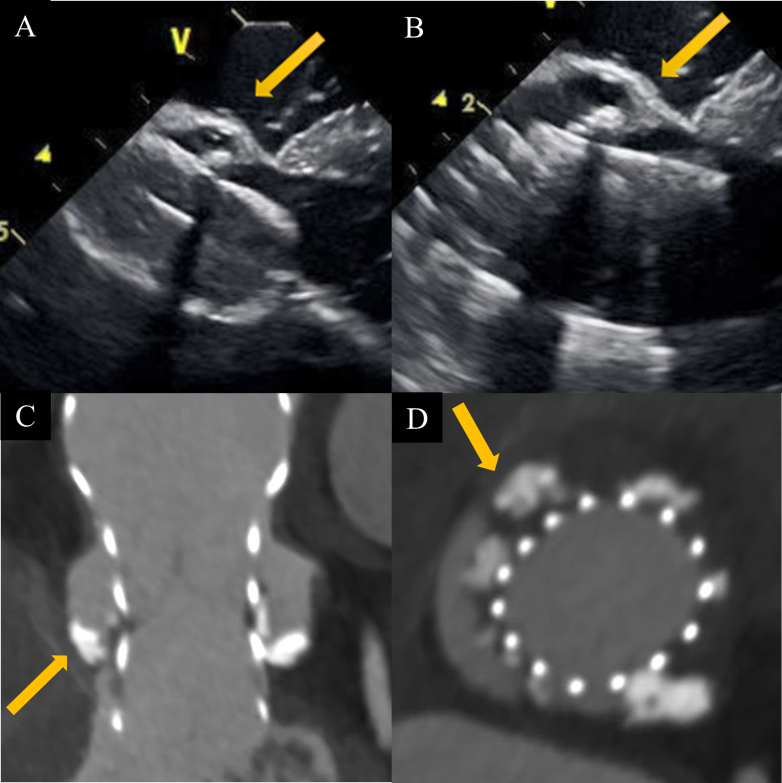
Calcification Between Bioprosthetic Valve and Membranous Septum Demonstrated by Intracardiac Echocardiography and Computed Tomography (A) Intracardiac echocardiography (ICE) image showing the calcification between the balloon and the membranous septum (MS) during predilation. (B) ICE image showing the calcification between the Evolut valve and the MS after valve deployment. (C and D) Computed tomography showing the calcification between the Evolut valve and the MS.
